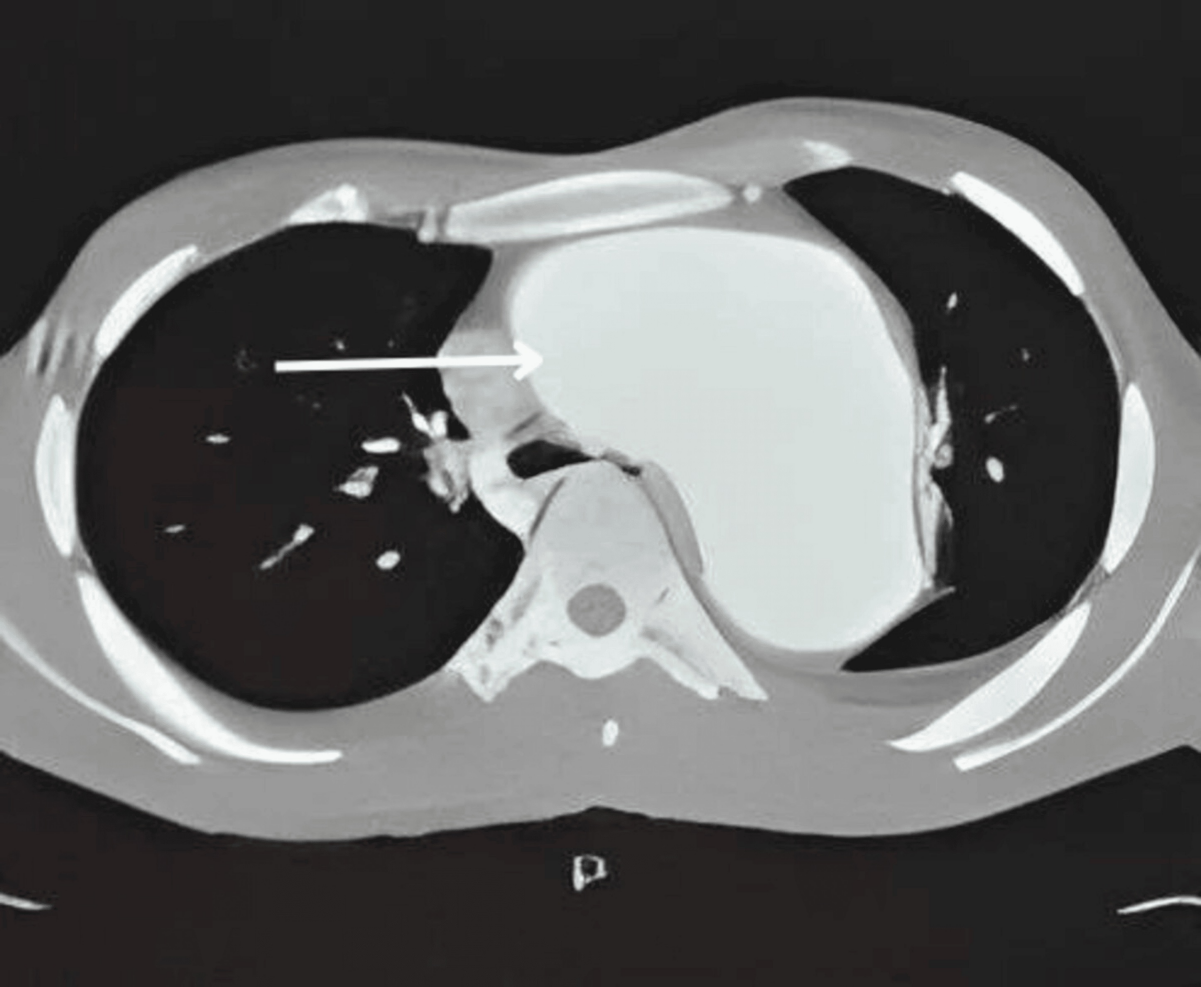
Axial chest computed tomography showing a large fusiform aortic aneurysm involving the ascending aorta, arch of the aorta, and proximal part of the descending aorta.
